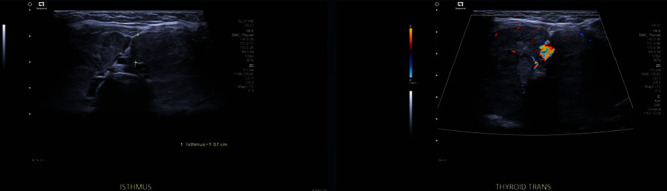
Ultrasound scan of the thyroid shows a markedly enlarged diffusely heterogenous thyroid gland without well-defined nodules.
